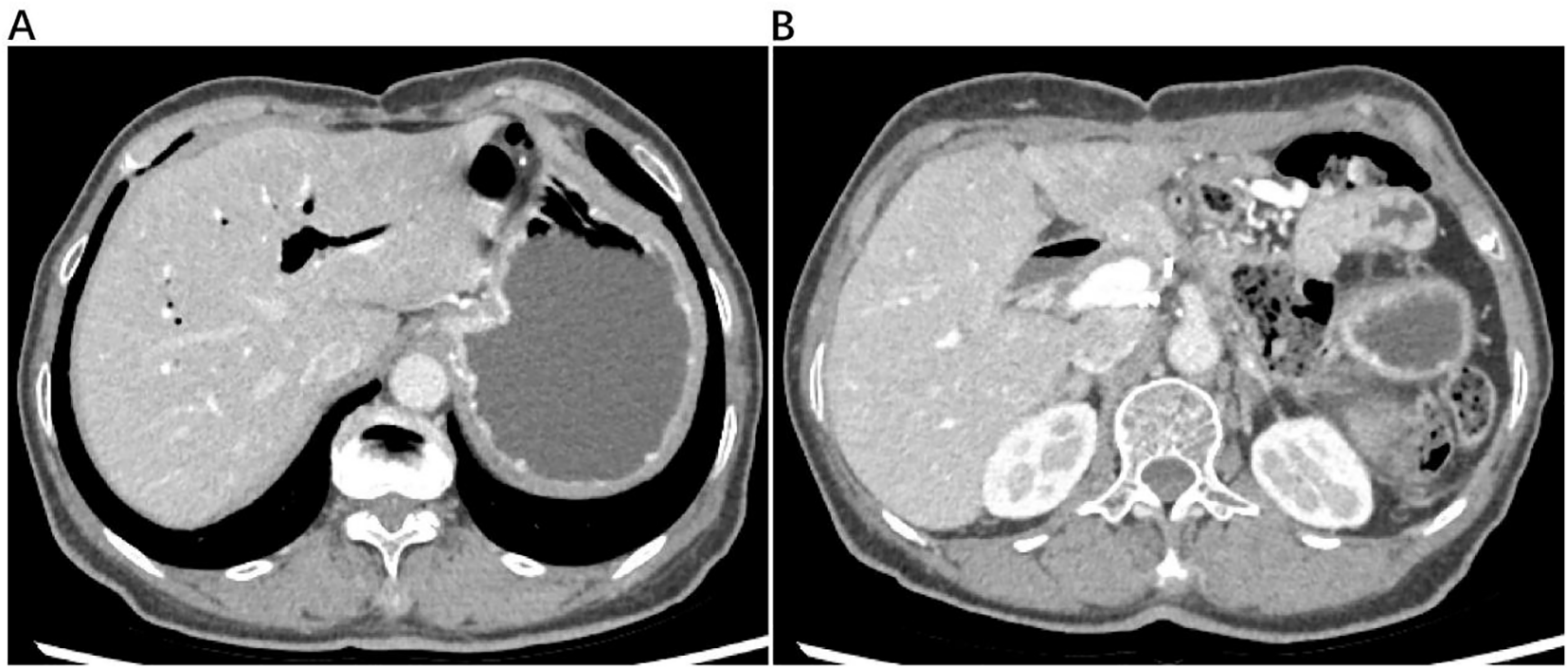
An abdominal CT scan, obtained for routine follow-up on June 12, 2024, demonstrated partial dilation of the intrahepatic bile ducts with pneumobilia (A). The CT scan revealed no radiological evidence of tumor recurrence (B).
